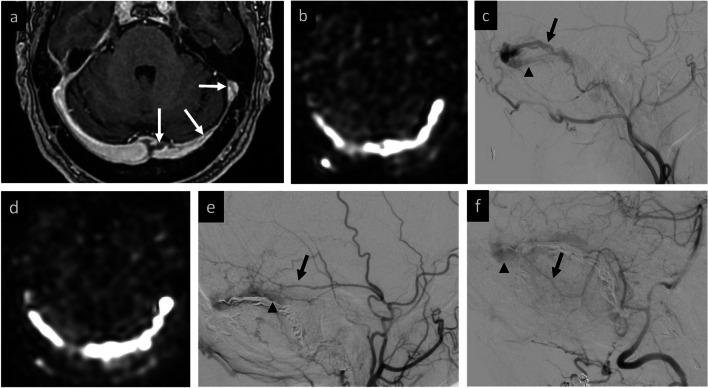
Concordant ASL and DSA findings with persistent transverse-sigmoid sinus dAVF after embolization. Baseline MRI: linear, non-occlusive thrombus in the torcular and left transverse sinus (white arrows, a) with marked venous ASL signal in the bilateral transverse sinuses compatible with AV shunting (b). Baseline DSA: lateral projection shows dAVF with dominant external carotid arterial (ECA) feeder (black arrow) and early venous drainage (black arrowhead) (c). Post-embolization MRI: persistent ASL signal in the transverse sinuses (d). Post-embolization DSA: Recruitment of additional ECA feeders (black arrows) and persistent early venous drainage (black arrowheads) confirm persistent shunt flow (e, f)
